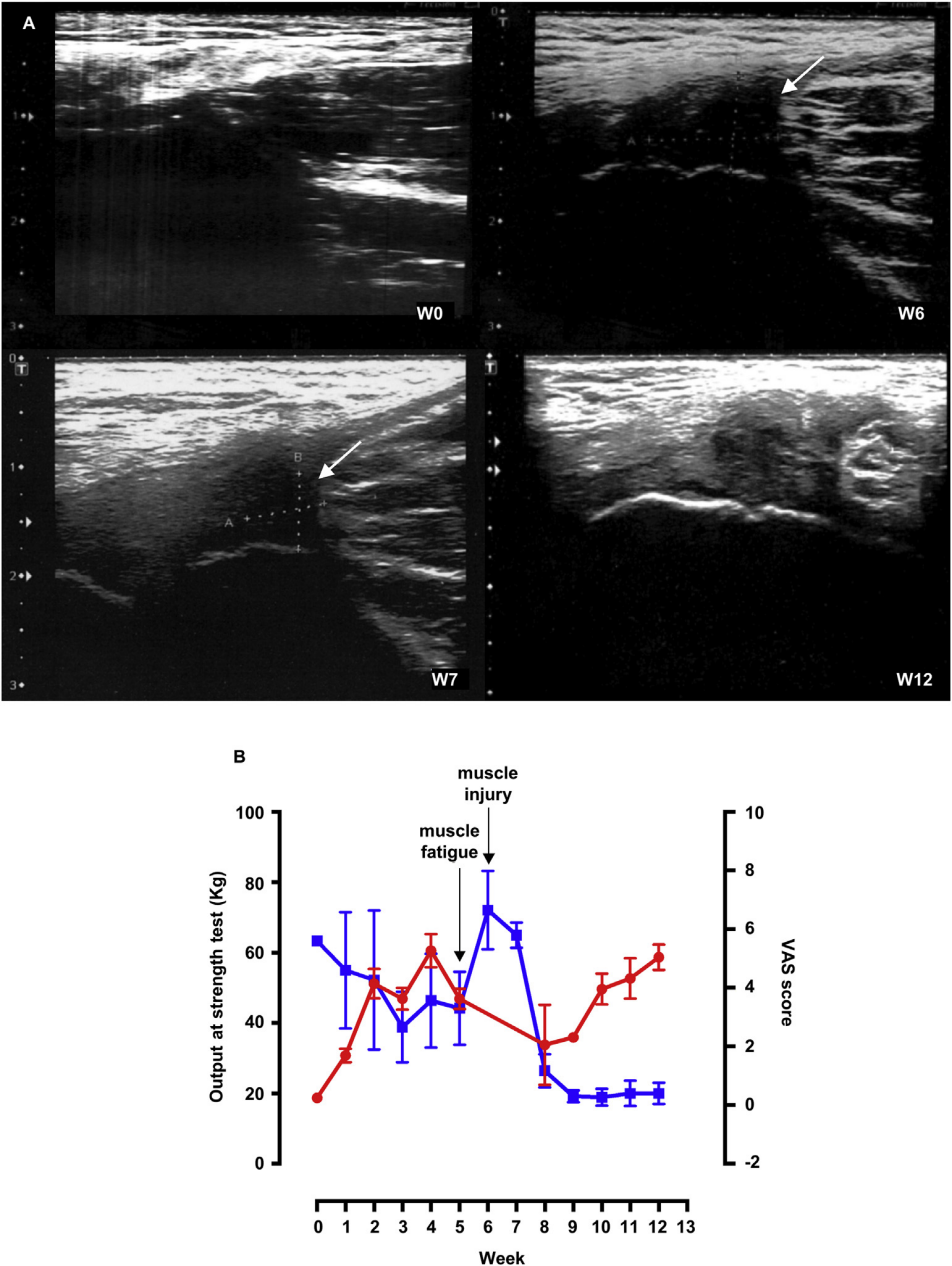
Case-report of traumatic injury. A) Ultrasound examinations at week (W)0, at the time of the injury (W6), one week after the injury (W7) and at the end of the treatment (W12). At W6 an edema is visible, which is already reduced after 1 week and is barely visible at W12 with no residual sign of injury. B) Strength and pain evaluations of the professional roller hockey player who had a traumatic injury. kg, kilogram; VAS, visual analogue scale.
